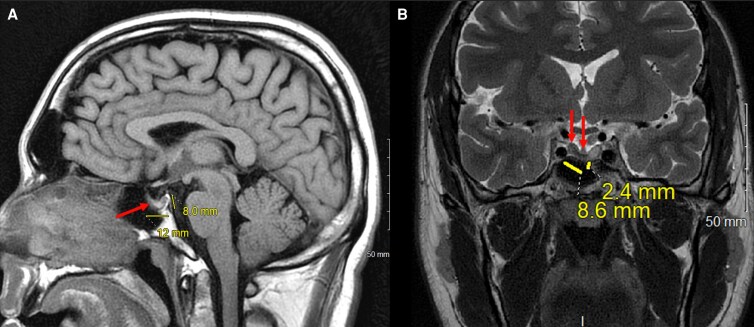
Subsequent magnetic resonance imaging of the brain with and without IV contrast. (A) Sagittal T1 view. (B) Coronal T2 view. The cyst (arrows in Fig. 2A) has decreased in size with mild TI hyperintensity focus. Hemorrhagic or proteinaceous debris is more clearly defined (identified by the arrows in Fig. 2B), and this can be a result of autoinfarction or involution of an adenoma consistent with response to treatment.
